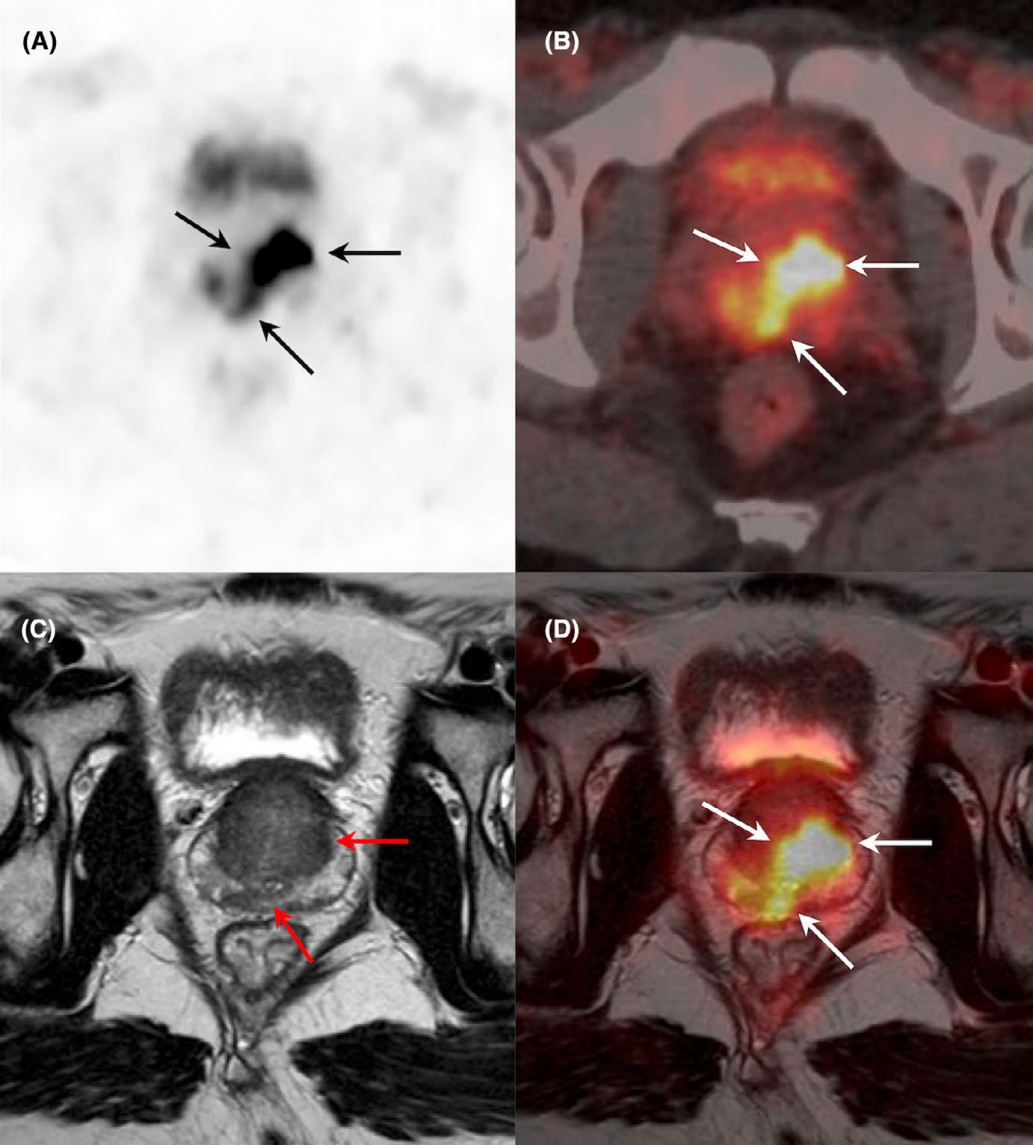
Initial staging in patient with biological suspicion of prostate cancer (PSA: 53.4 ng/mL). [^18^F]PSMA1007 PET/CT and MRI imaging (axial PET (A), PET/CT (B), T2‐weighted MRI (C), PET/MRI fused images (D)) demonstrated intense pathological uptake in the bilateral peripheral zone, mostly involving the left anterior, posterolateral and posteromedial zones, with invasion of the seminal vesicle (MRI PI‐RADS: 5, SUVmax: 20.8). [^18^F]PSMA1007 PET also showed tumoral extension in the transitional zone, which was not revealed by MRI. Pathological examination after biopsy confirmed the presence of acinar adenocarcinoma with lymphovascular invasion and Gleason score: 7 (3 + 4).
